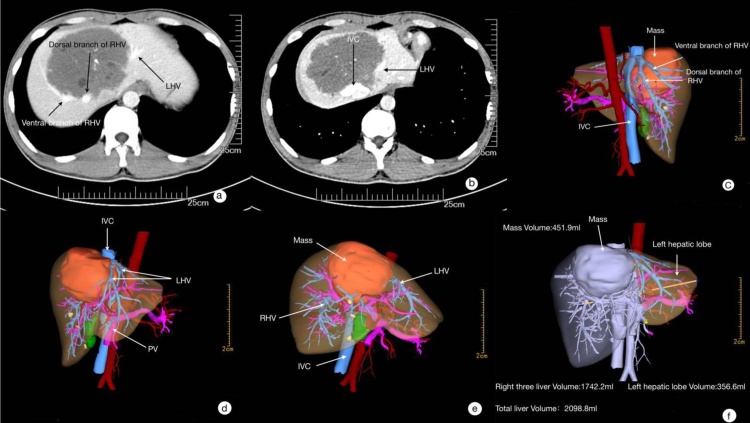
Enhanced abdominal CT and 3-dimensional (3D) imagings analysis. a: the lesion invaded the root of right hepatic vein; b: invaded the root of left hepatic vein; c: invaded the root of right hepatic vein branch (ventral/dorsal branches) and the retrohepatic anterior wall of IVC; d: invaded the root of left hepatic vein (3D image); e: the lesion was adjacent to the porta hepatis (3D image); f: the volume of the left liver section (3D image).
